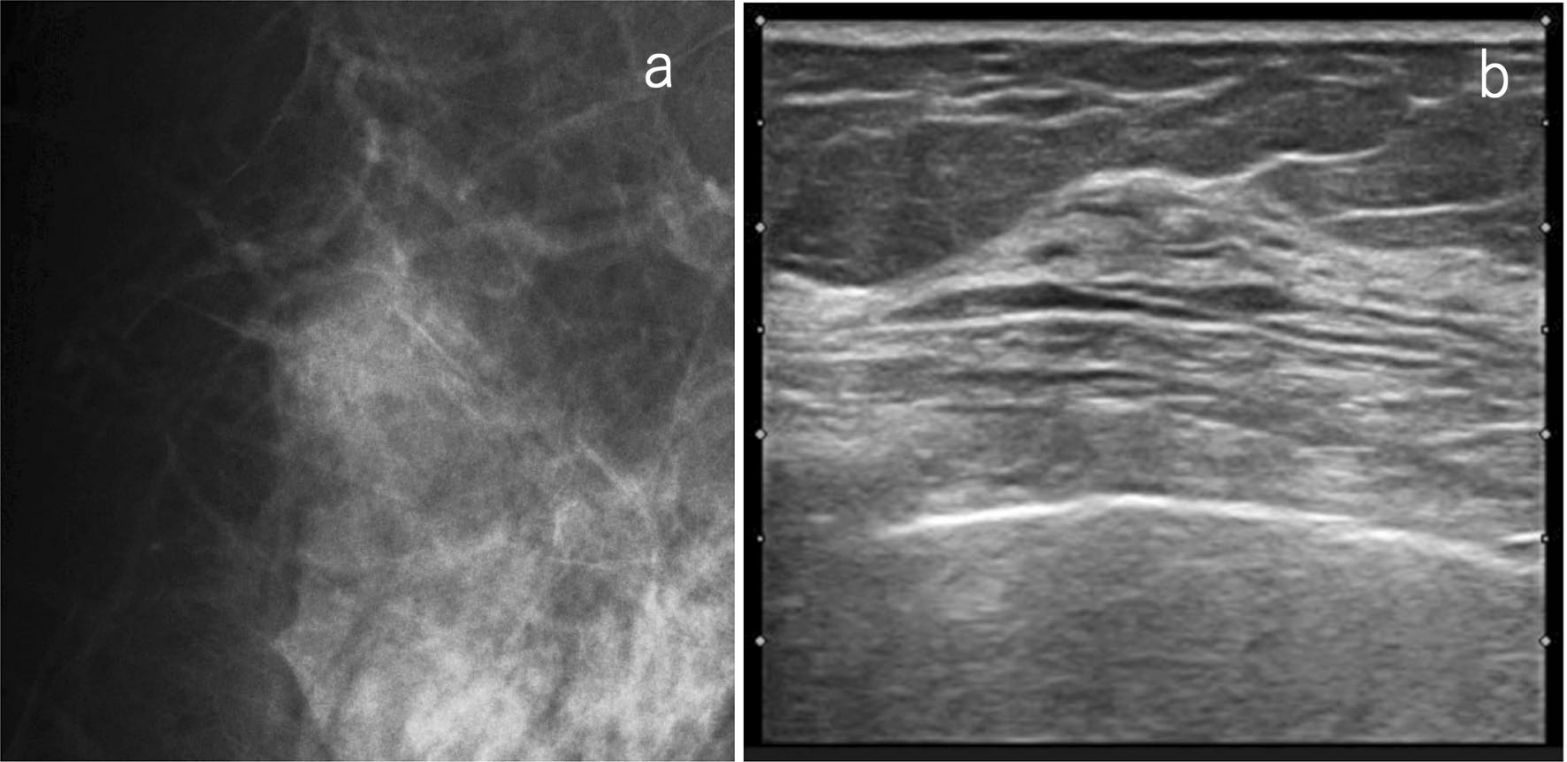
A case of focal density on mammography. a Mammography: focal density, SMC 3. b Ultrasound: focally thickened normal mammary glands were detected as abnormal findings on MG, SUC 1. The overall assessment category: SC 1 (*SMC* screening mammography category, *SUC* screening ultrasound category, *SC* screening category)
